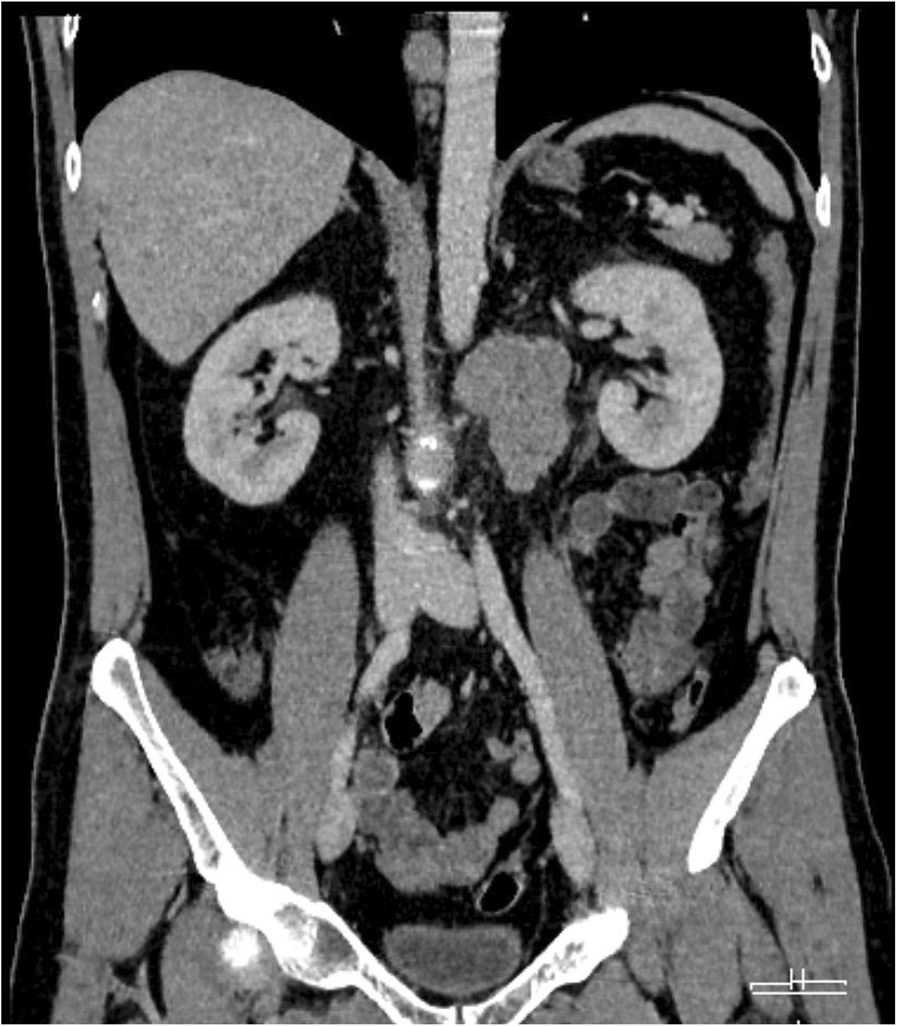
Abdominal computed tomography, coronary section view showing the large retroperitoneal metastasis of seminoma before initiation of chemotherapy.
